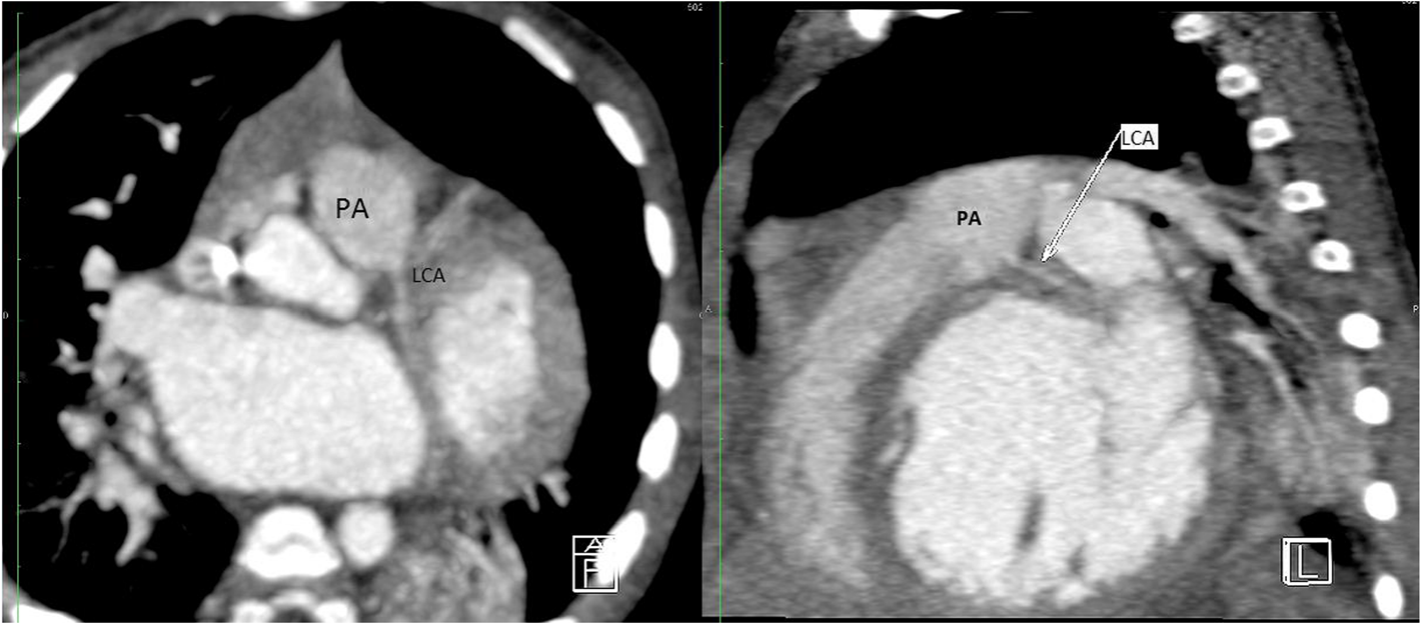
Computed tomography angiography MultiPlan. Left coronary artery emerging from the pulmonary artery trunk. LCA: Left coronary artery. PA: Pulmonary artery.
